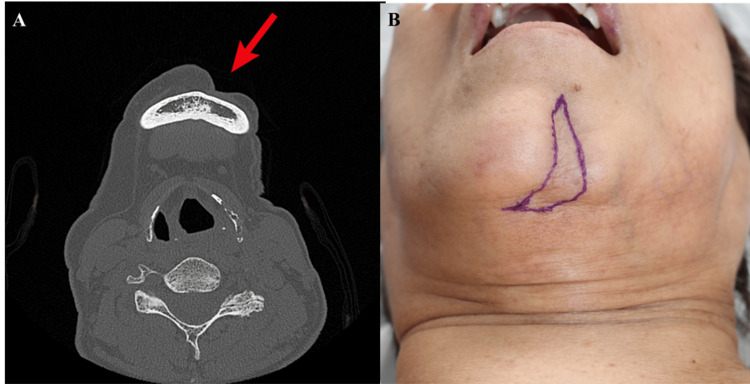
Preoperative imaging and planning (A) Axial CT showing soft tissue atrophy without bony involvement. (B) Skin marking, outlining scar excision, and flap design.
